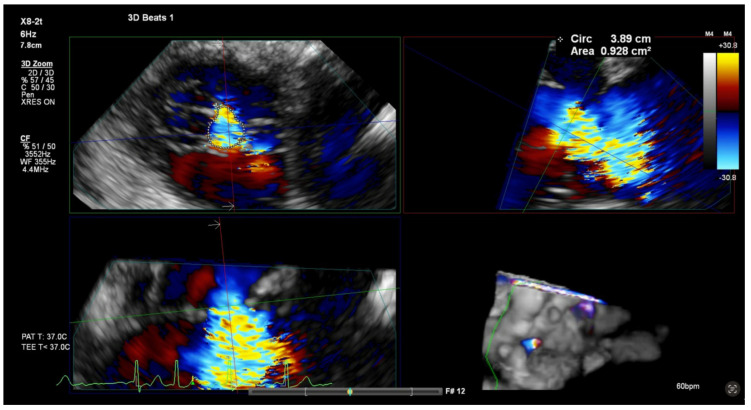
3D-TEE MPR to measure the VCA. After locking the orthogonal planes, the green plane is positioned at the level of the VC width in the red and blue plane. After careful adjustment, the VCA can be visualized and can be measured by direct planimetry in the green box. TEE, transesophageal echocardiography; MPR, multiplanar reconstruction; VC, vena contracta; VCA, vena contracta area.
